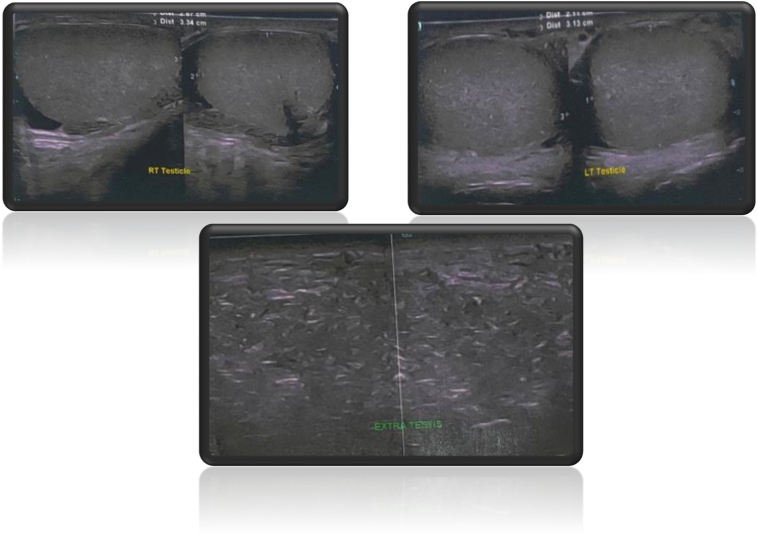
Ultrasonography of scrotum and intrascrotal mass.
